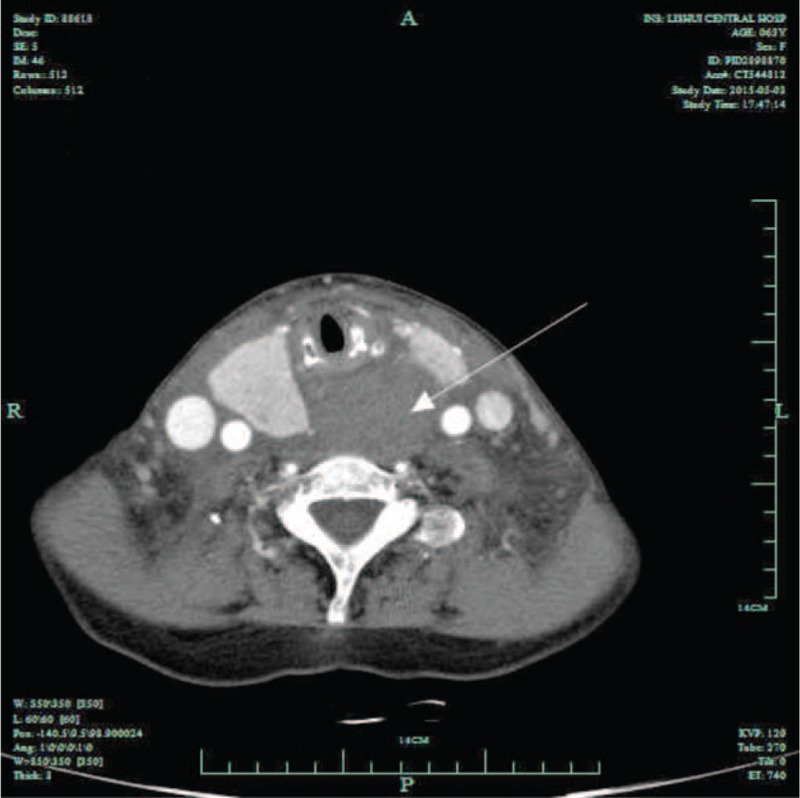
CT scans demonstrated the mass had enlarged and was nonenhancing.
